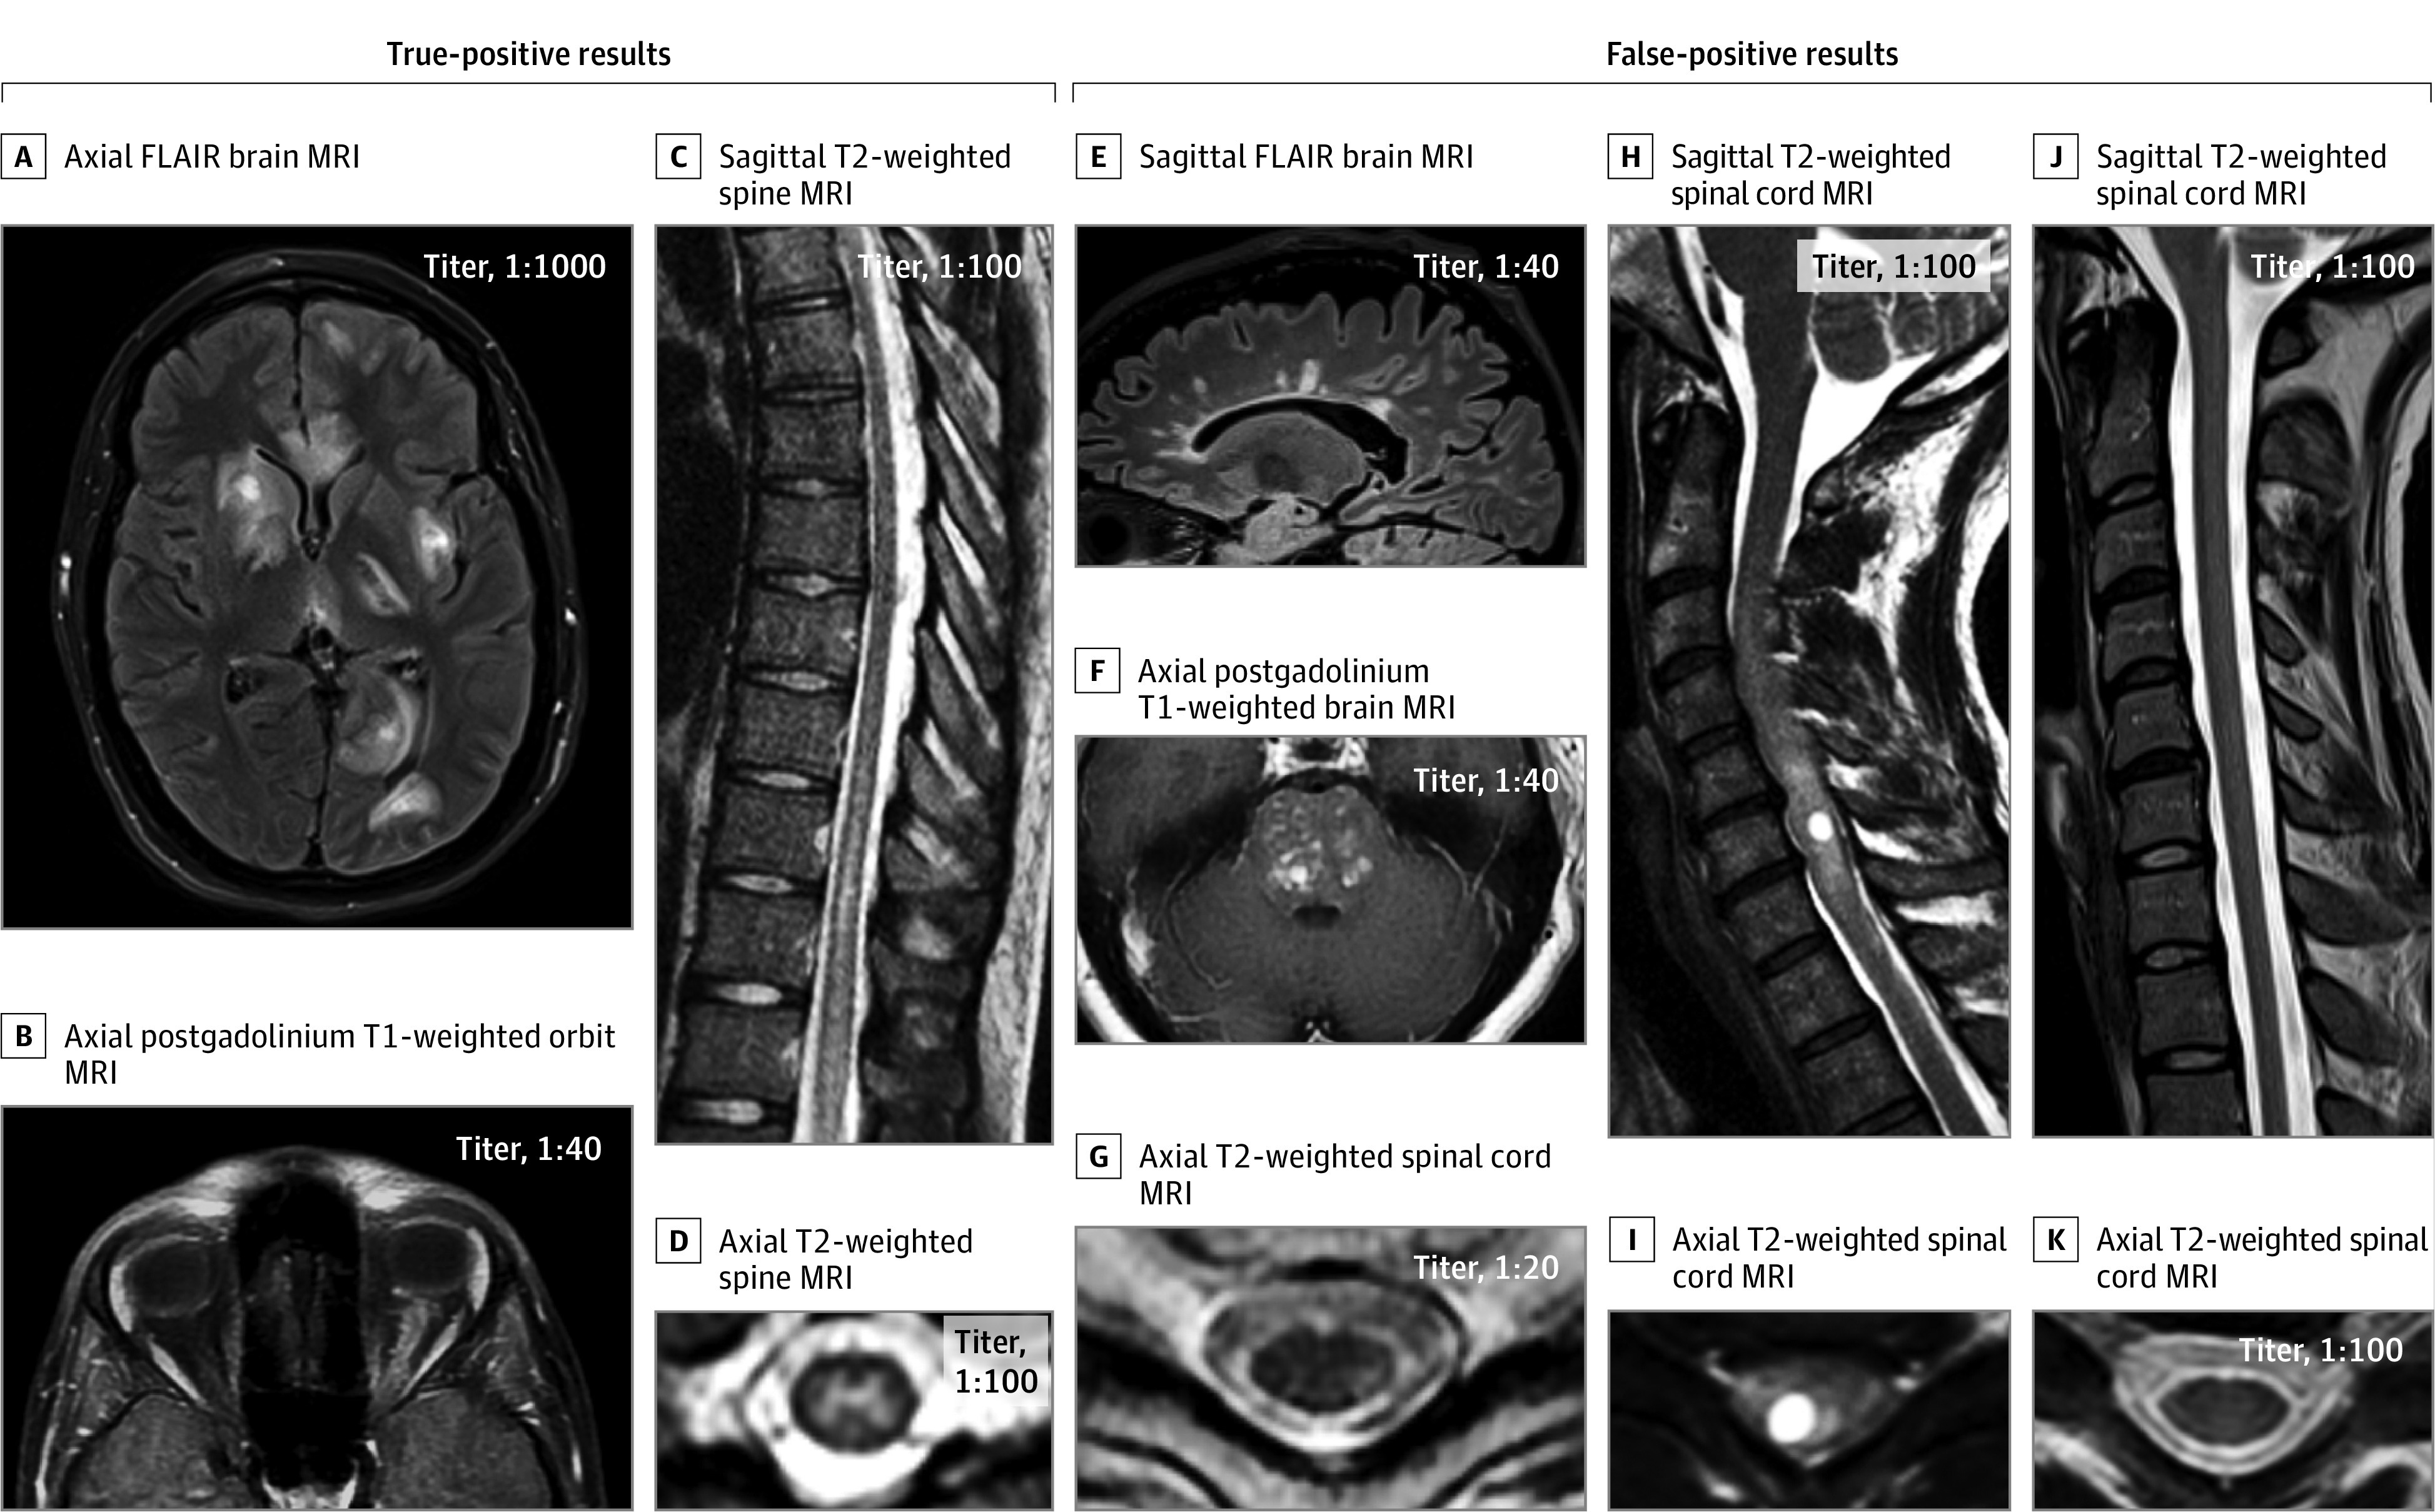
Representative Examples of Magnetic Resonance Imaging (MRI) Findings in True-Positive vs False-Positive Myelin Oligodendrocyte Glycoprotein (MOG)–IgG1 Cases True-positive cases (A-D): axial fluid-attenuated inversion recovery (FLAIR) image showing large, multifocal, poorly demarcated lesions on brain MRI in a patient with an acute disseminated encephalomyelitis attack in MOG-IgG1–associated disorder (MOGAD) (A); axial postgadolinium T1-weighted orbit MRI showing longitudinally extensive enhancement of the left optic nerve sheath in a patient with optic neuritis as a manifestation of MOGAD (B); sagittal T2-weighted images showing a longitudinally extensive myelitis lesion along the lower thoracic spinal cord, with predominant involvement of the central gray-matter on axial images in MOGAD (C and D). False-positive cases (E-K): sagittal FLAIR image showing Dawson-finger T2-hyperintense lesions perpendicular to the ventricle, typical of multiple sclerosis (E); axial postgadolinium T1-weighted images showing multiple areas of nodular enhancement in the pons that brainstem biopsy confirmed to be a histiocytic disorder (F); axial T2-weighted image showing a peripheral dorsolateral hyperintense lesion abutting the surface of the spinal cord, in another patient with multiple sclerosis (G); sagittal T2-weighted image showing a faint longitudinally extensive T2-hyperintense lesion accompanied by marked cervical spinal cord swelling with an intralesional cyst (H), also appreciable on axial images (I), which biopsy confirmed to be a glioma; sagittal (J) and axial (K) T2-weighted spinal cord MRI showing normal signal intensity and initial atrophy in a young adult man with X-linked adrenomyeloneuropathy.
